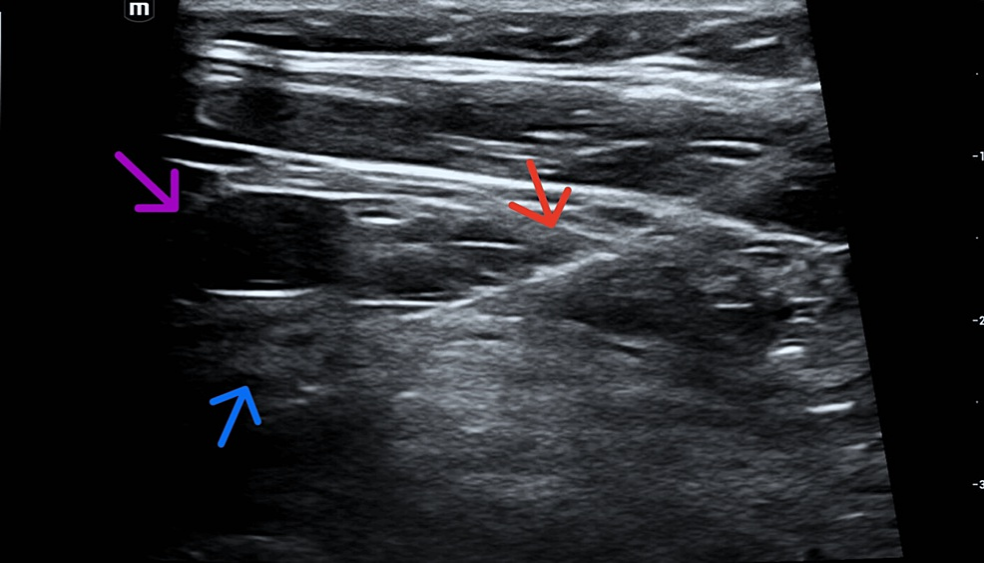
Ultrasound still image of stellate ganglion catheter placement. Red arrow: Tuohy needle and catheter; blue arrow: stellate ganglion; purple arrow: carotid artery.
